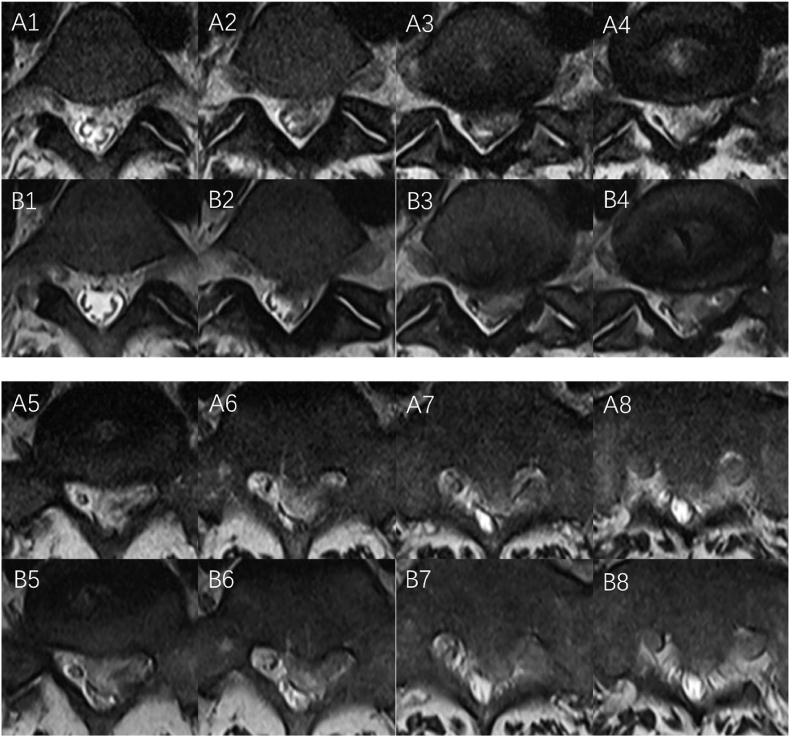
(MRI, Axial images of motion segment L5/S1 (T2-Signal): A1-A8: Images after the injury, from cranial to caudal: giant epidural ventral sequestration in the spinal canal. The sequestration in image A4-A6 showed a slightly lower T2-signal in comparison to CSF. B1–B8: Images by follow-up one month after the injury, from cranial to caudal The images showed a further decrease in T2-signal compared to the MRI after the injury. The decreased T2-signal of the sequestration in comparison with CSF was more significant (A3/B3, A4/B4, A5/B5, A6/B6). The compression on Dura was slightly reduced (A4/B4, A5/B5, A6/B6, A7/B7, A8/B8).
